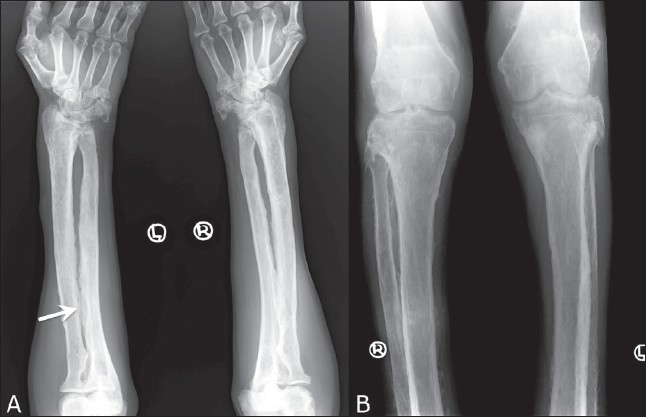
Anteroposterior radiographs of both forearms (A) and upper legs (B) show symmetric, shaggy periosteal proliferation extending up to the epiphyses, with expansion of the radius and ulna at the wrist and of the tibia and fibula at the knee, along with calcification of the interosseous membranes (arrow)
